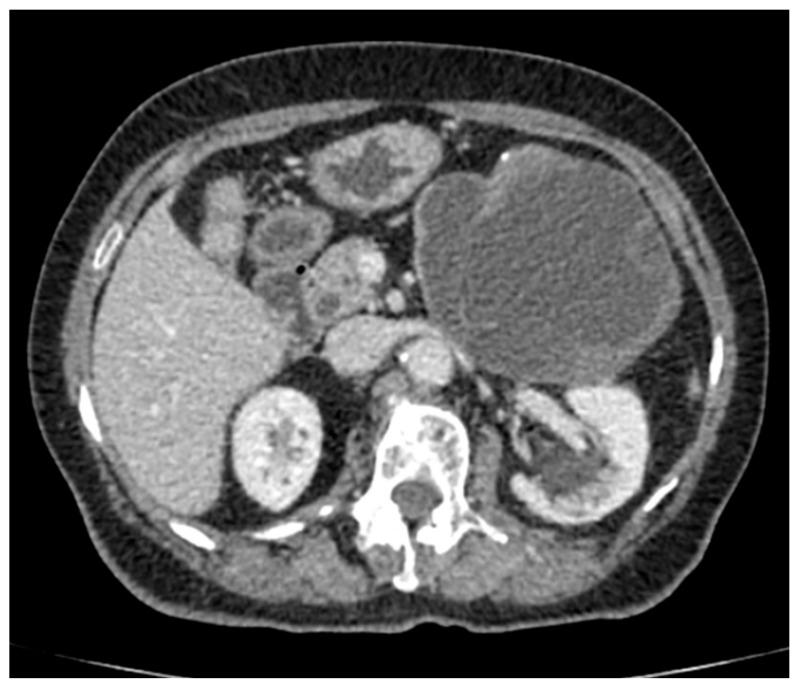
Axial section contrast-enhanced abdominal computed tomography (CT) shows a well-defined round to oval cystic lesion 116 × 100 mm large in the tail of the pancreas, causing compression of the stomach, with well-defined peripherally enhancing margins, and an irregularly thickened wall. Discrete internal septation and peripheral microcalcifications are also detected.
